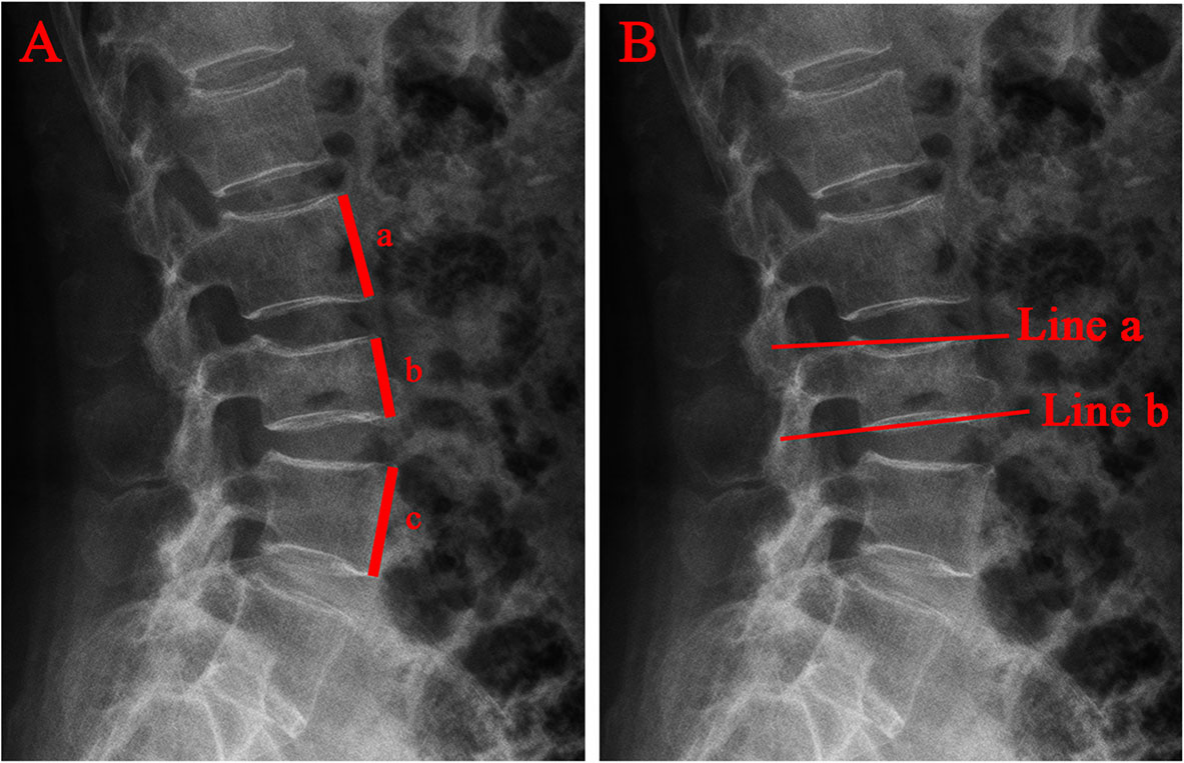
Radiographic evaluation of compressed vertebrae. A The anterior vertebral height ratio(AVHR) was defined as the height of the anterior wall of the compressed vertebral body(b) / (the height of the anterior wall of the upper vertebral body(a) + the height of the anterior wall of the lower vertebral body(c))×2. B Cobb angle was defined as the angle formed by the upper endplates(Line a) and lower endplates(Line b) of the fractured vertebral body
